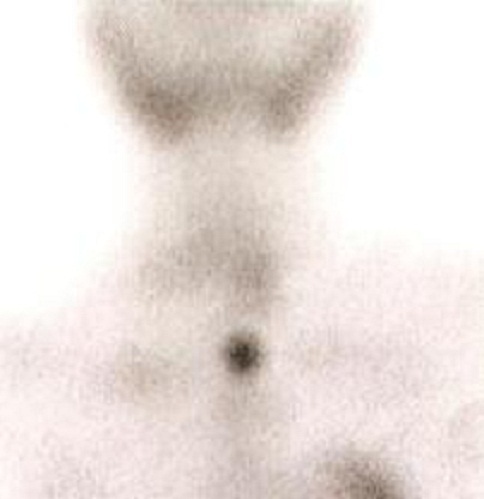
Sestamibi parathyroid scintigraphy revealed an increased focal uptake
